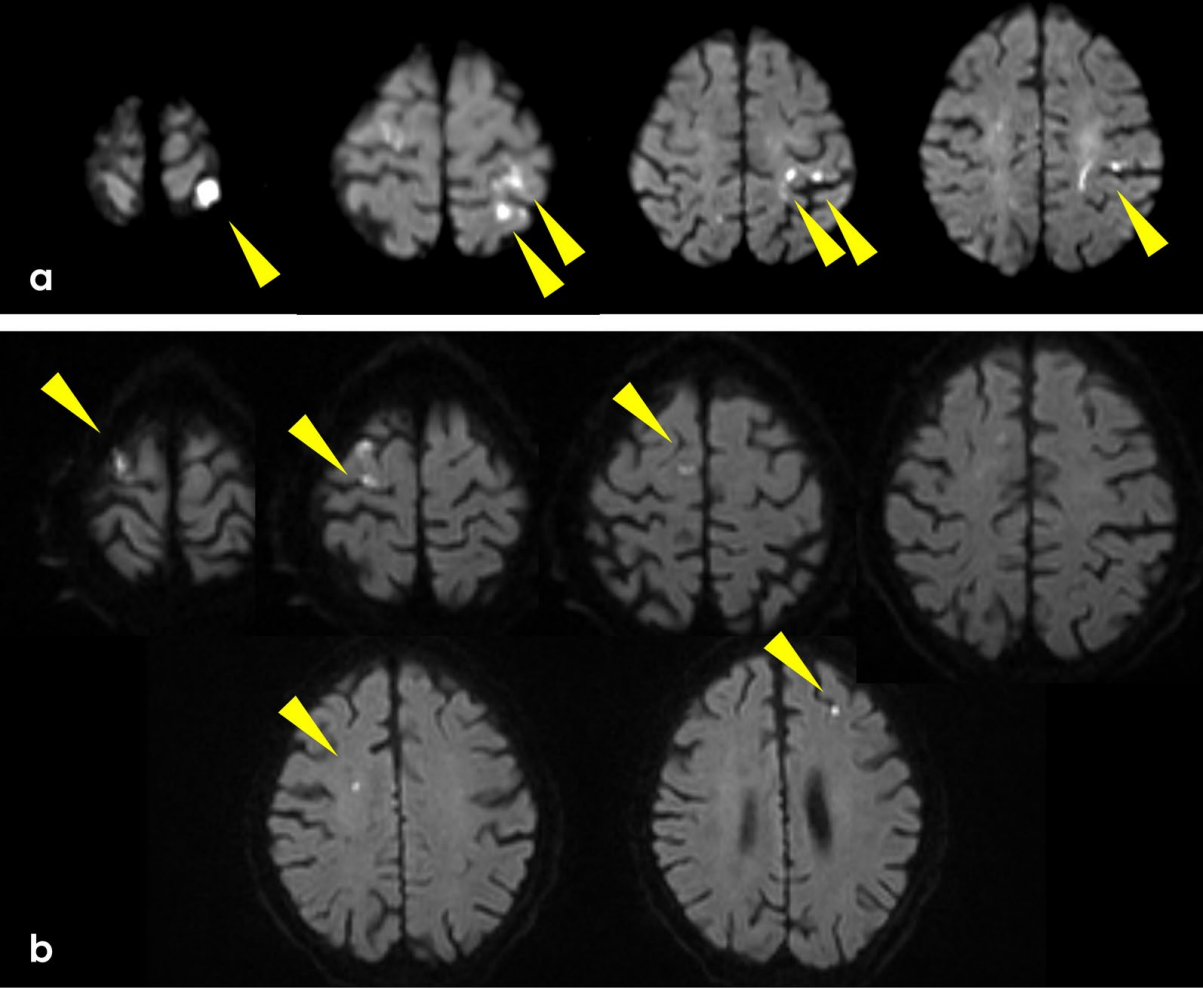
Diffusion-weighted magnetic resonance imaging at a the initial cerebral infarction and b the second cerebral infarction. a Multiple foci of restricted diffusion in the left parietal lobe. b Many areas of restricted diffusion in the bilateral lobes
